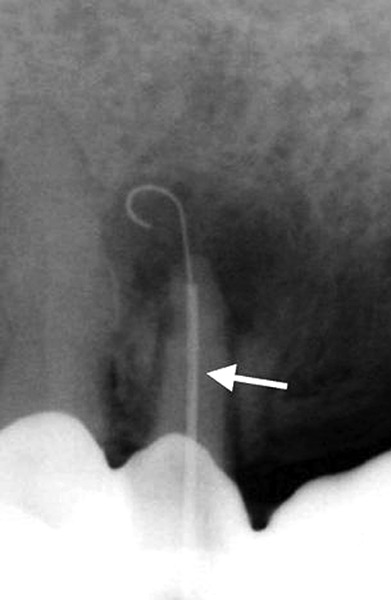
The Apexum NiTi Ablator in a periapical lesion. The NiTi Apexum Ablator fully extruded from its sheath (arrow) and into the periapical lesion
